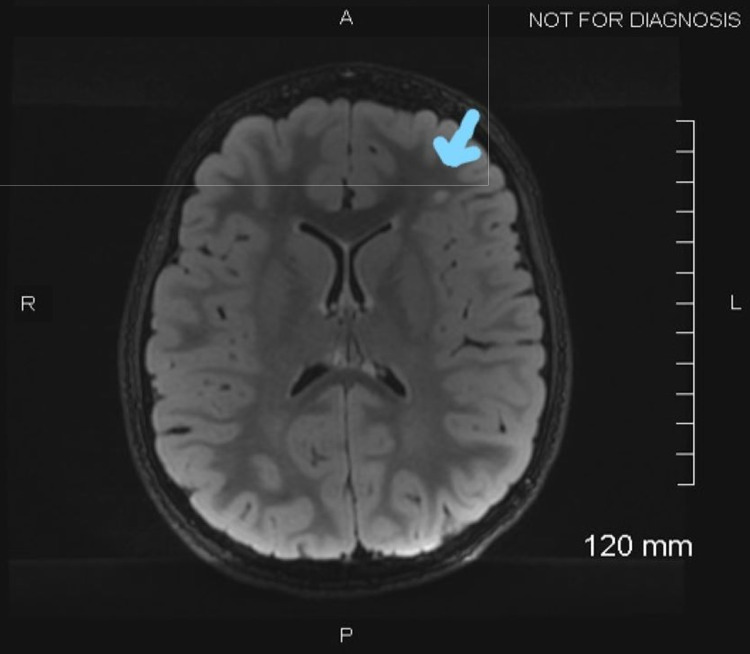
MRI of the brain showing T2 fluid-attenuated inversion recovery (FLAIR) multifocal subcortical white matter changes.
